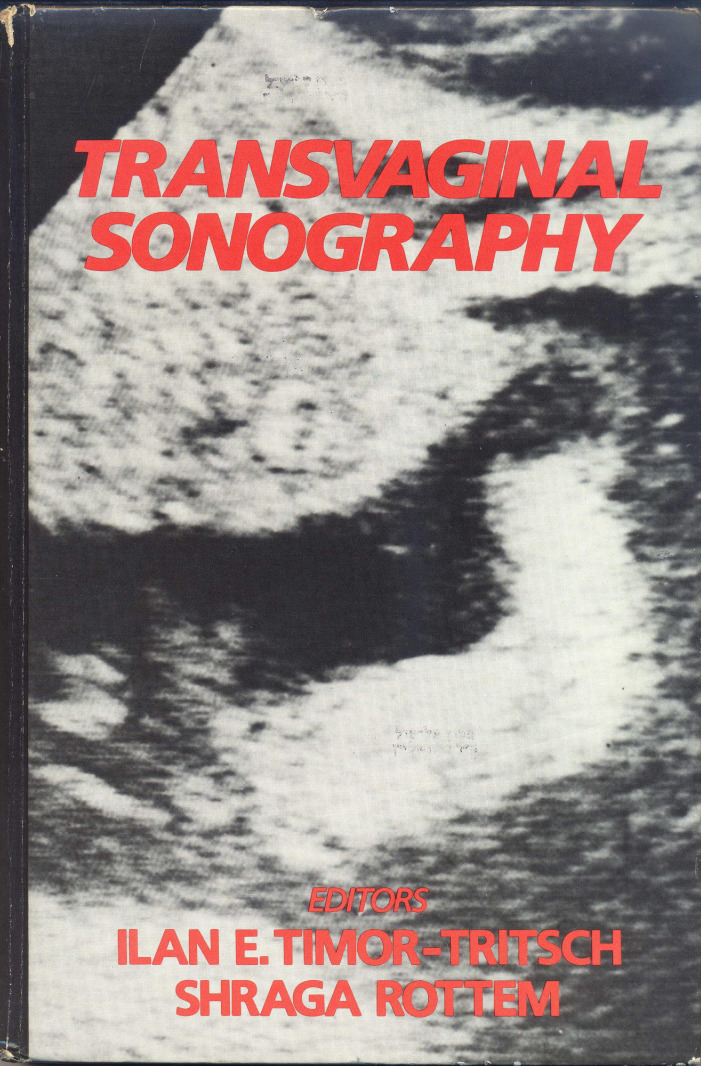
The First Edition of the Book *Transvaginal Ultrasound* by Ilan E. Timor-Tritsch and Shraga Rottem (1987).
